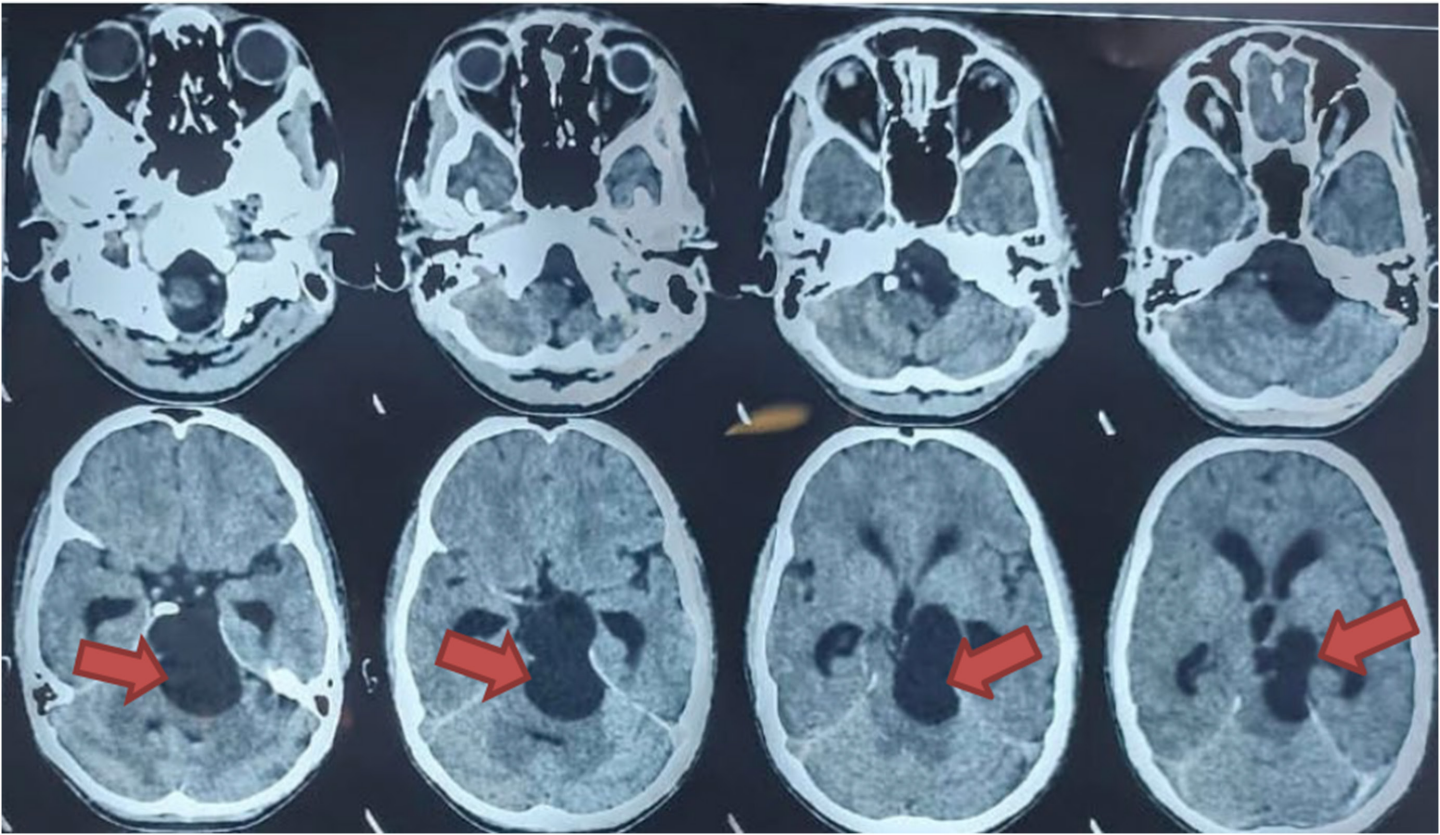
Computed tomography (CT) imaging with findings suggestive of a giant arachnoid cyst causing communicating hydrocephalus.
